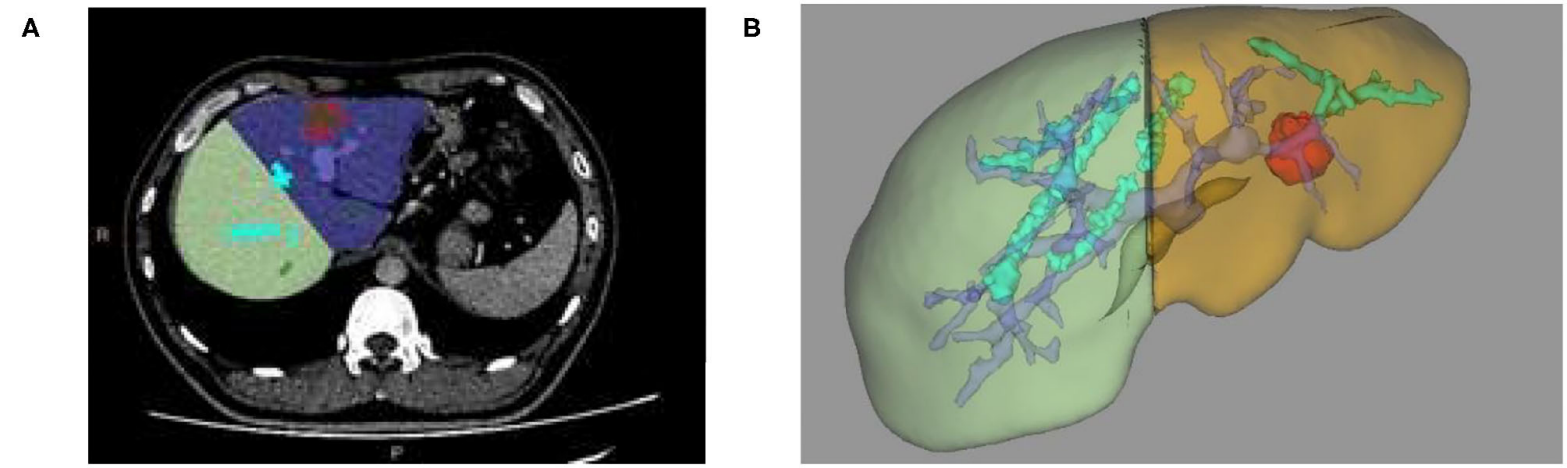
(A) Two-dimensional CT enhanced image, (B) 3D reconstruction based on liver segmentation and tumor growth model on CT data.
